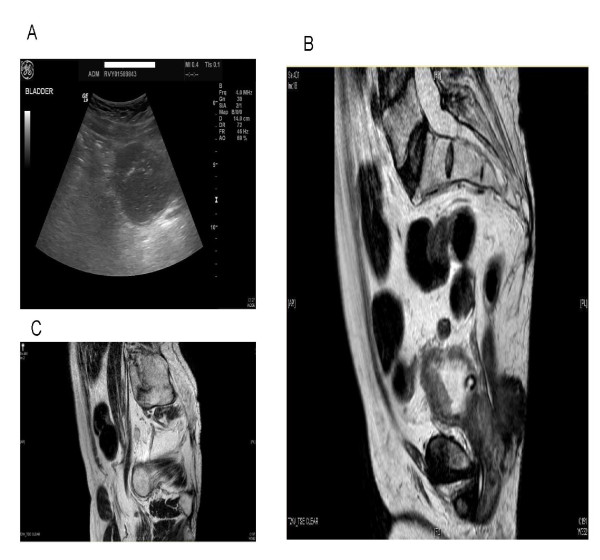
Ultrasound scan and MRI of pelvis. A Ultrasound scan of urinary bladder (23 July 2010): 150mls sterile saline instilled into the urinary bladder via suprapubic catheter: No gross bladder abnormality was demonstrated. Small amount of debris noted within the bladder. B MRI of pelvis performed on 29 October 2010 shows 24 mm thick anterior bladder wall tumour involving the full thickness of the bladder wall. There is streaking of the peri vesical fat anteriorly consistent with extravesical extension. C MRI of pelvis performed on 29 October 2010 showed 15 mm × 10 mm left iliac lymph node. No other pelvic lymphadenopathy was seen.
